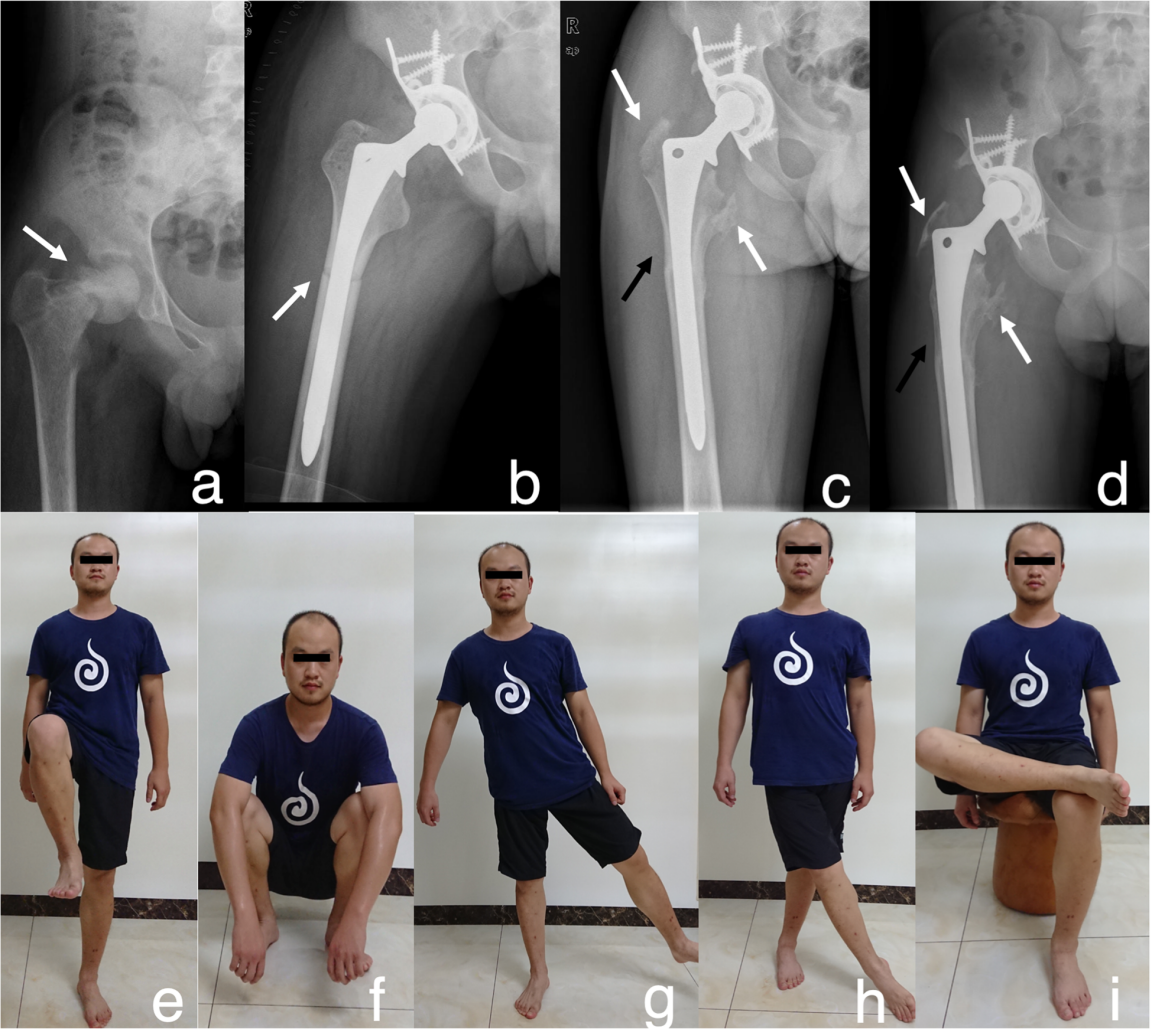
Patient (No. 13) with the diagnosis of OS in the right femoral neck. a White arrow indicated pathological fracture occurred during the chemotherapy pre-operation. b The immediate radiograph after the reconstruction with uncemented APC. c-d 12 months and 96 months post-operation: white arrows indicated the avulsion of the trochanteric fractures, while the black arrow indicated the resorption of the allograft bone. e-i The function of the hip, 96 months post-operation
